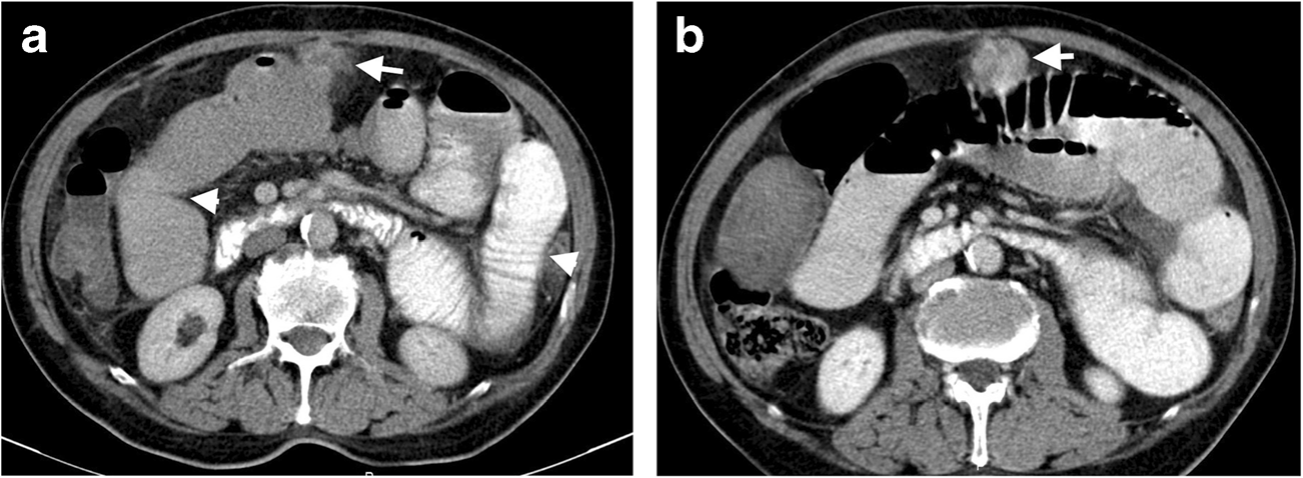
Intestinal obstruction in a 79-year-old male secondary to diverticulitis. a Axial contrast-enhanced CT of a 75-year-old man presenting with abdominal pain and distention reveals a diverticulitis with the appearance of a inflamed diverticulum and pericolonic fat stranding (*arrow*) in the transverse colon. Bowel segments proximal to the localization of diverticulitis is dilated. b Axial CT performed 2-months after the first CT reveals thickening of the colonic wall (*arrow*) leading to intestinal obstruction
